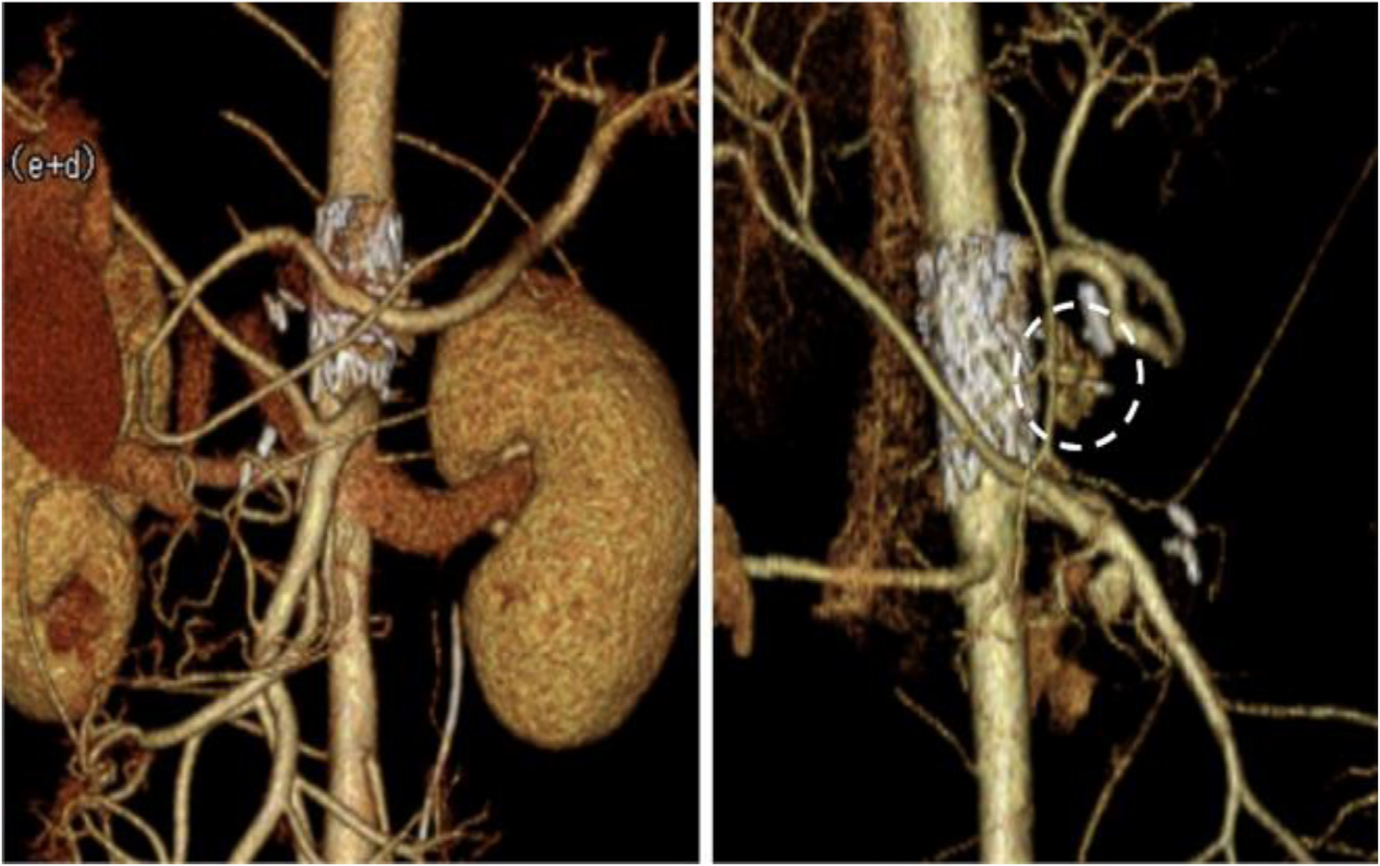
A postoperative computed tomography (CT) scan revealed that the celiac artery aneurysm (CAA) was treated at its origin and had disappeared *(dotted circle)*. Blood flow in the celiac artery branches was maintained by the pancreaticoduodenal artery arcade.
